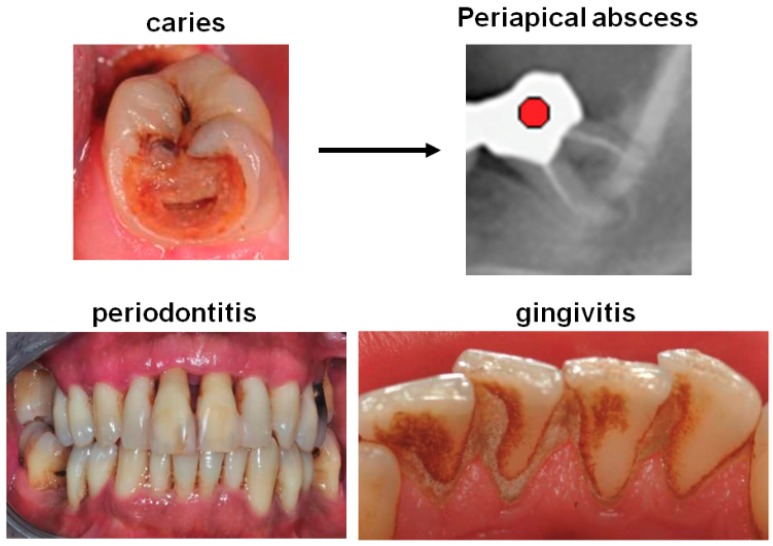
Oral inflammation affecting the teeth, gingiva, and the periodontium. Shown are examples of a tooth affected by severe caries, a tooth with abscess formation revealed by orthopantomography, a denture with severe periodontitis with evidence of extensive loss of attachment and gingival recession at most teeth, and an example of gingivitis with extensive calculus formation and a swollen and reddened inflamed gingiva.
